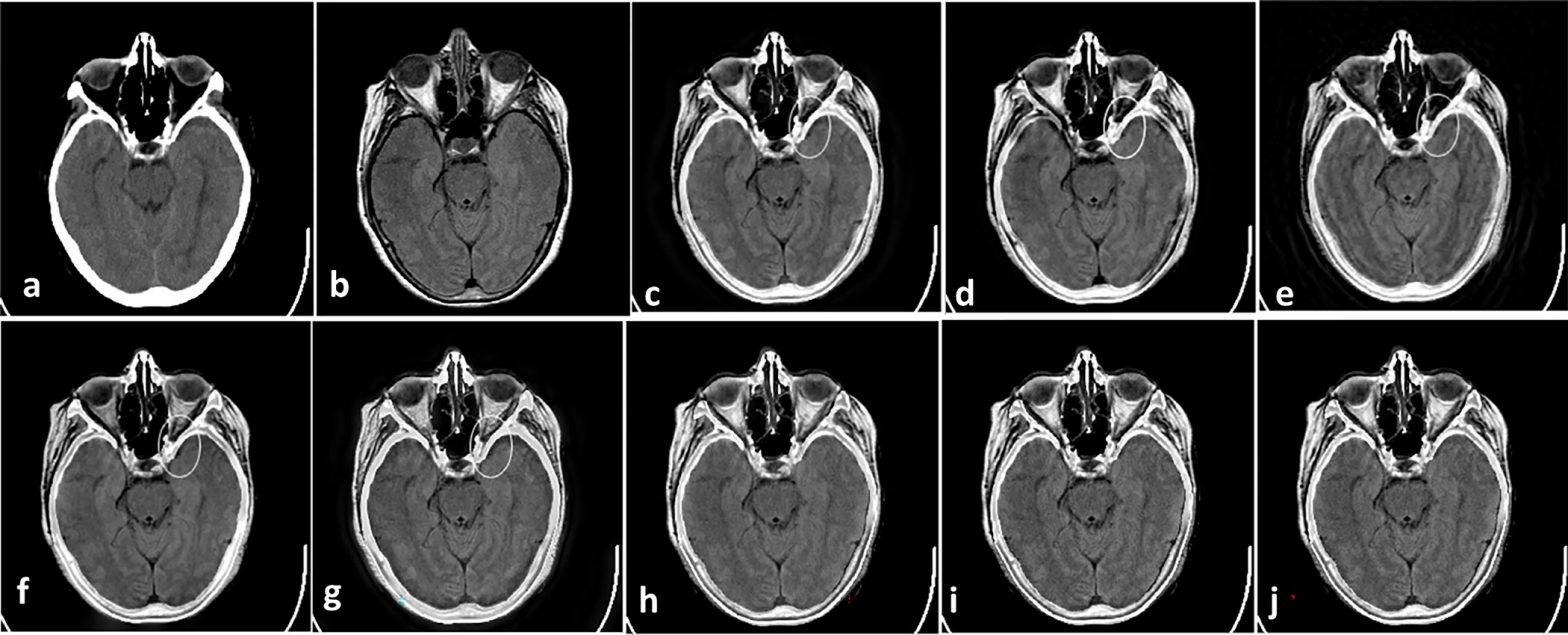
Experiment 7 [53]: Results of fusing CT and MR images of a brain. (a) CT image; (b) MR image; (c) NNSST [93]; (d) FMSAP [95]; (e) CST [106]; (f) ST-NSST [96]; (g) MFDF-NSST [53]; (h) the proposed method with PCA, (i) max, and (j) weighted averaging model, respectively.
